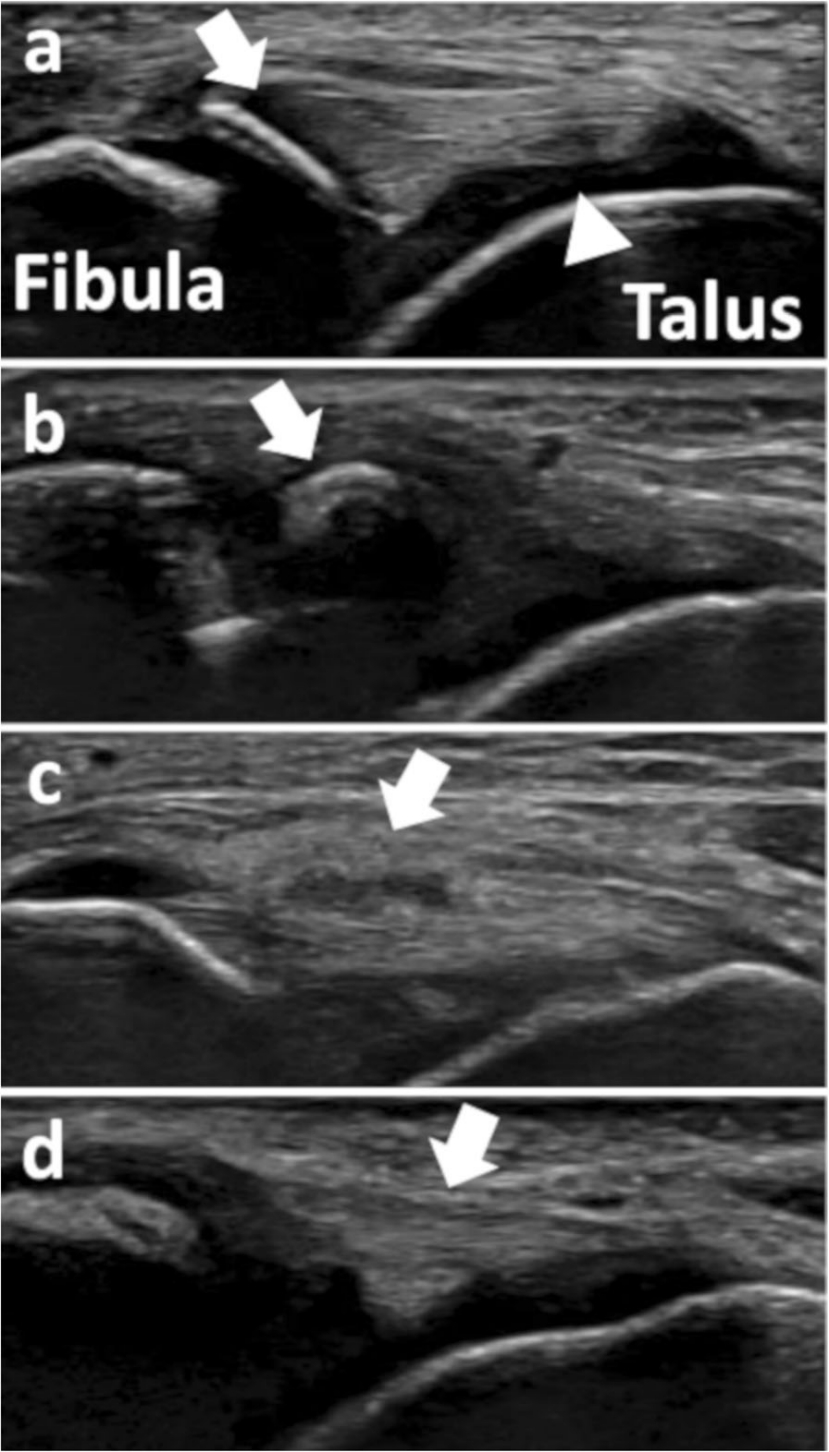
Sonographic image of the longitudinal view of the anterior talofibular ligament. a Avulsion fracture (arrow) at the fibular insertion of the anterior talofibular ligament (arrowhead), b Subfibular ossicle (arrow), c Anterior tibiofibular ligament injury (arrow), d No osseous or ligamentous injury (arrow)
